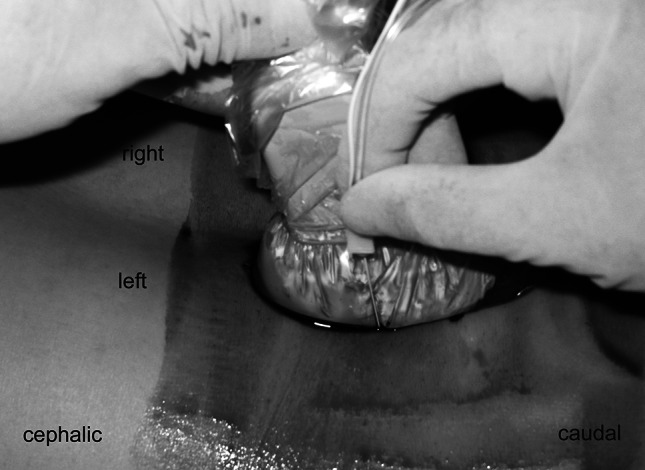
The position of patient, the needle, and the probe of the ultrasound apparatus under the left S1 nerve root block. The needle is pointed slightly medially from the lateral side of the probe and advanced toward the target with ultrasound guidance
